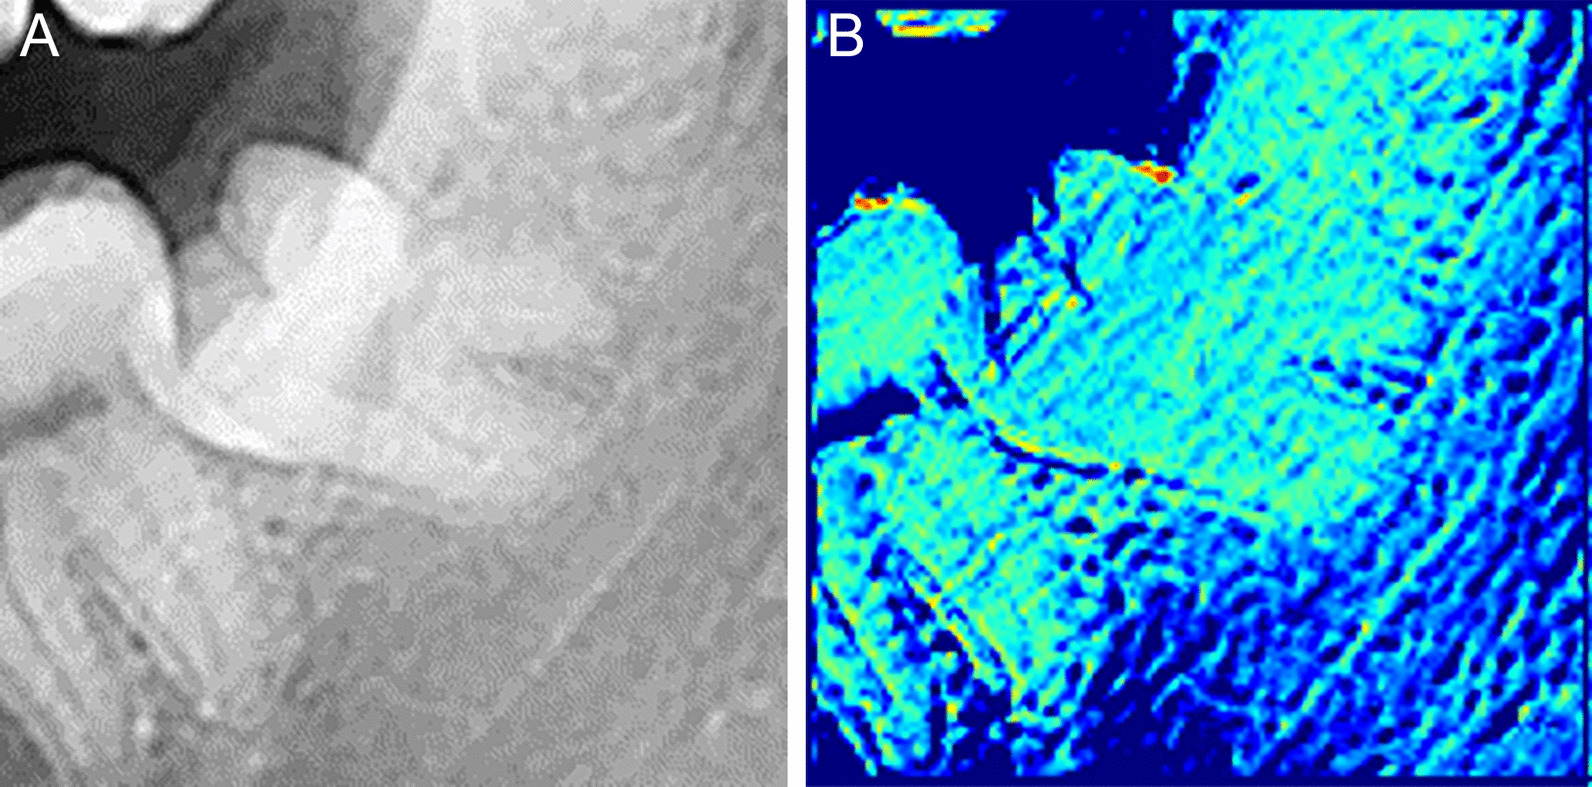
a, b Gradient-weighted Class Activation Mapping (Grad-CAM) was used to visualize which part of the panorama X-ray was referenced by the CNN model of this study when making a prediction
